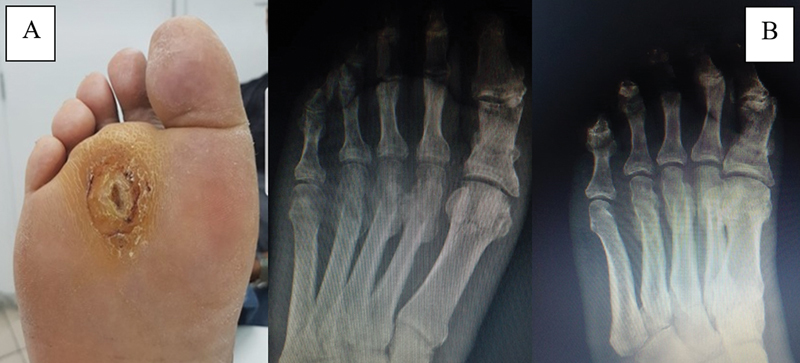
Clinical and radiographic appearance of the right foot in the third month after surgery. (
